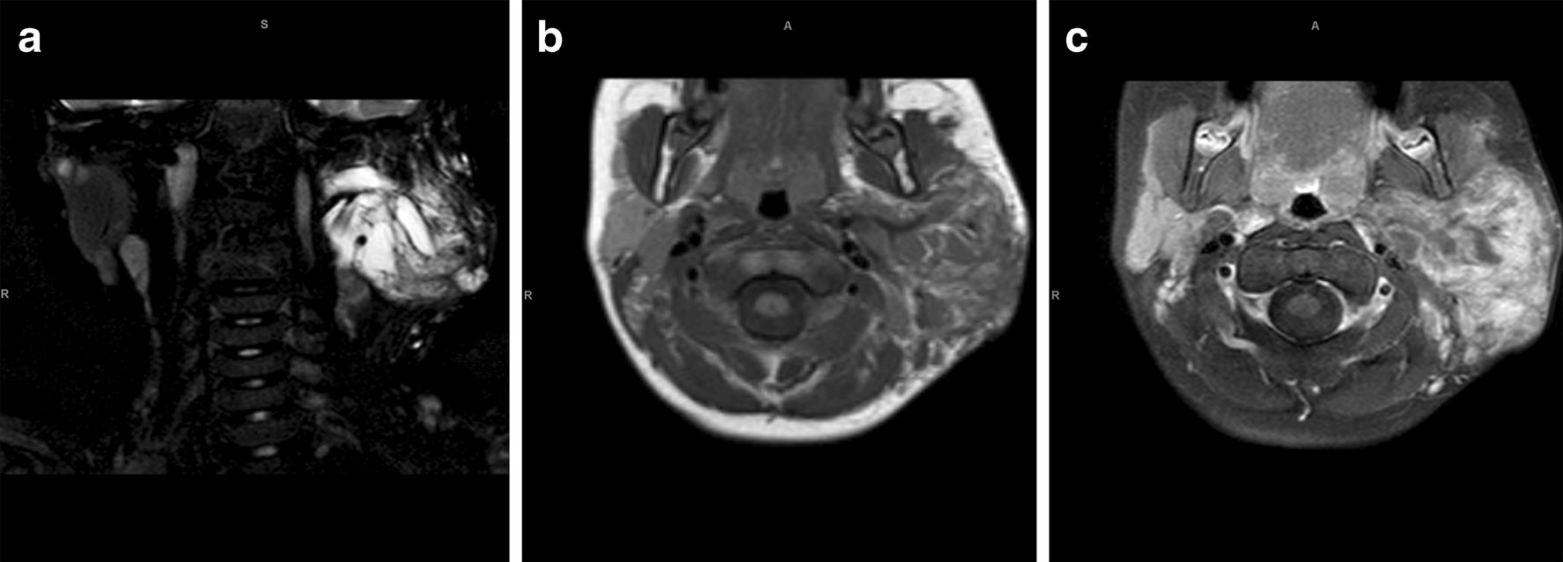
Parotid hamartoma. Coronal T2-W MRI (a) shows the left parotid gland to be expanded and almost entirely replaced by a heterogeneous, mixed solid and cystic lesion which is mixed intensity on the axial T1-W view (b) and enhances avidly post-contrast (c)
